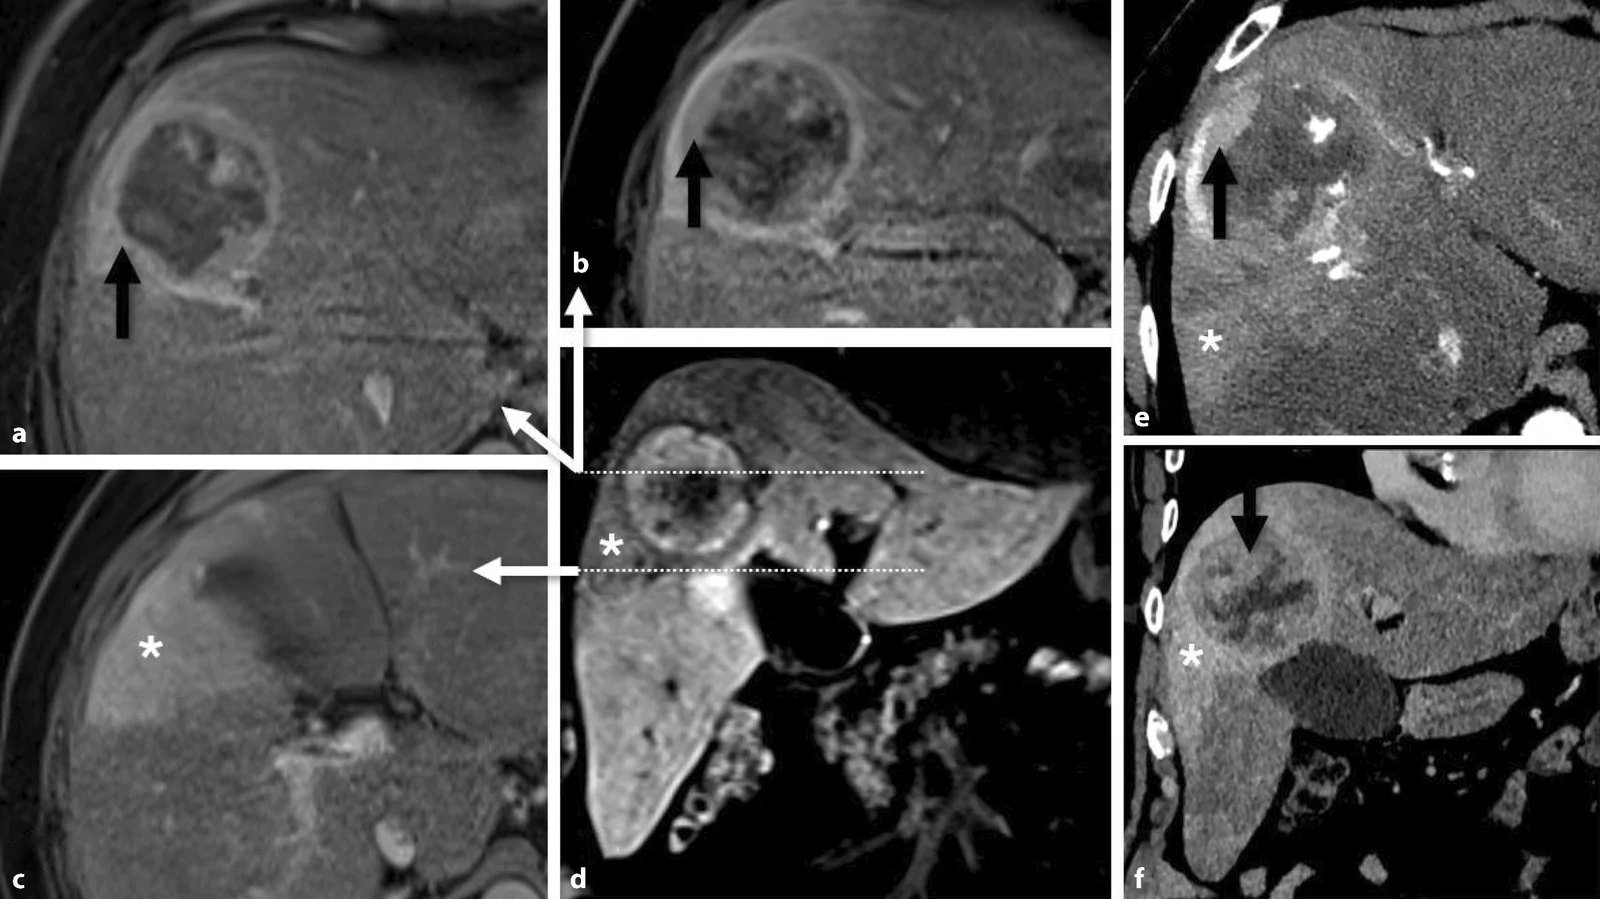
a–d Leber-MRT und e, f -CT. In der Leber-MRT unter Verwendung des hepatospezifischen Kontrastmittels (Gadoxetsäure; Primovist^TM^) zeigt sich intraläsional in der Leberraumforderung im Segment VIII (a, b obere Ebene, gestrichelt) in der a arteriellen Phase ein zirkuläres randständiges Kontrastmittel-Enhancement (*Pfeil*). Dieses weist b ab der portal-venösen Phase ein Wash-out-Phänomen auf (*Pfeil*) bei angrenzender schmaler Pseudokapsel sowie d mit einem in der hepatobiliären Phase detektierbaren Primovist^TM^-Uptake. Angrenzend an die Leberraumforderung (c untere Ebene, gestrichelt) zeigt sich peritumoral eine flächige arterielle bis portal-venöse Hyperperfusion mit d in der Spätphase nach 20 min vermindertem Primovist^TM^-Uptake (*Stern*). Im CT e, f zeigen sich analog zur MRT e intraläsional eine randständige arterielle Hyperperfusion (*Pfeil*) mit ab der portal-venösen bis zur späteren Phase f geringem Wash-out-Phänomen (*Pfeil*) sowie zentral einzelne arterielle Tumorgefäße mit zunehmend protrahiertem Kontrastmittel-Enhancement (e, f). Peritumoral stellt sich ebenfalls ab der arteriellen Phase eine regionale Hyperperfusion im Lebersegment VIII und V dar (*Stern*), die bis zur Spätphase nach 4 min persistiert
